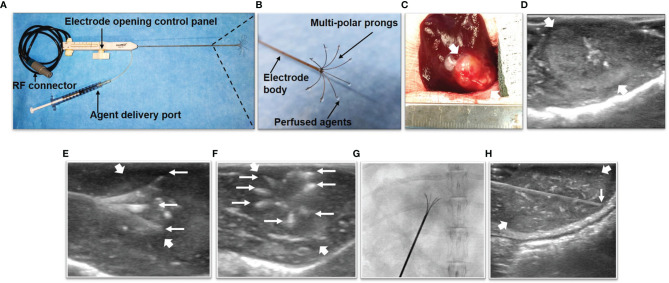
The multi-functional perfusion-thermal radiofrequency (RF) electrode, treatment process of liver tumor, and *in-vivo* interventional optical imaging process of liver tumor. (A) The multi-functional perfusion-thermal RF electrode with multipolar agent infusion prongs. Micro-thermosensors are equipped at the tips of the prongs for real-time measurement of RFH at approximately 42°C. (B) The details of RF electrode tip. (C) Morphology image of tumor (arrow) during surgery, which is detected by ultrasound imaging (D). The perfusion-thermal electrode is advanced into the center of the tumor (between large arrows) under real-time ultrasound guidance. (E) Longitudinal and (F) transverse sonographic images, showing the electrode prongs (small arrows). The electrode prongs (small arrows) are opened to the desired size array, further confirmed by X-ray image (G), to completely cover the tumor peripheral zone for sufficient delivery of the therapeutic agent. During delivery of LTX-401, RFH at the tumor is generated with the same device to further enhance oncolytic treatment. (H) Under real-time ultrasound imaging guidance, the micro-OI needle (small arrow) was advanced into the periphery of the tumor (between large arrows), to generate intratumoral “inside-output” optical images at different directions/points around the tumor periphery.
